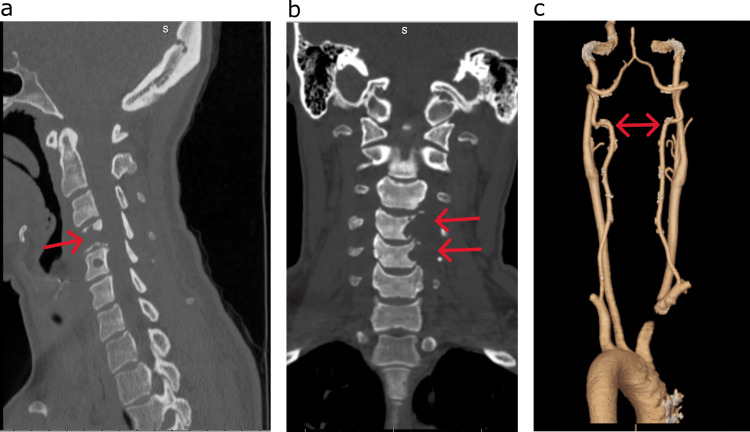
Pre-operative CT scans (a) Sagittal view demonstrating the left-sided C4-C5 erosive lesion (red arrow). (b) Coronal view demonstrating the left-sided C4-C5 erosive lesion (red arrows). (c) CT angiogram demonstrating the co-dominant vertebral arteries (red arrows). CT: computed tomography
